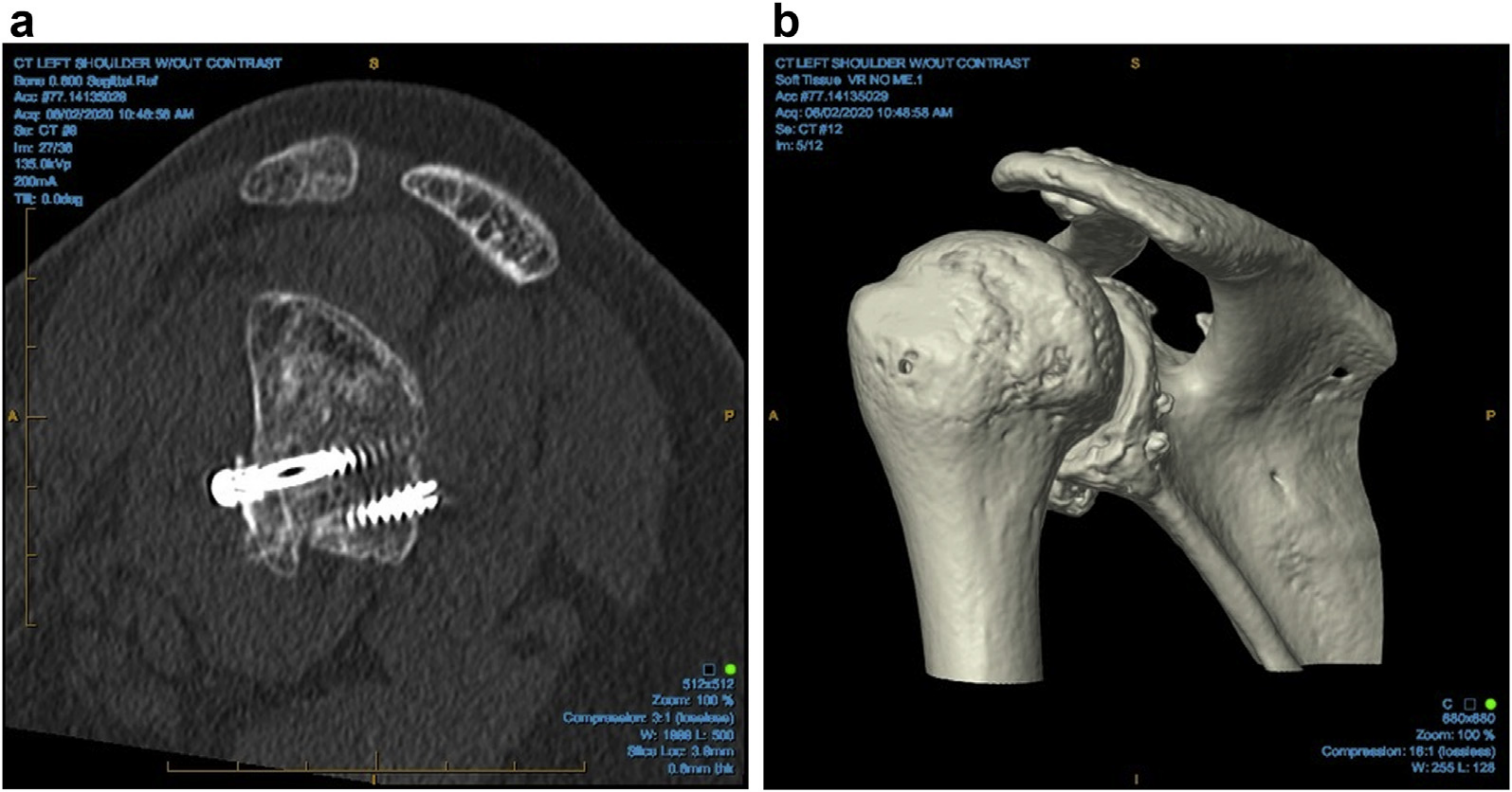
Bicortical screw fixation on (a) CT and (b) 4D CT scan. *CT*, computed tomography.
